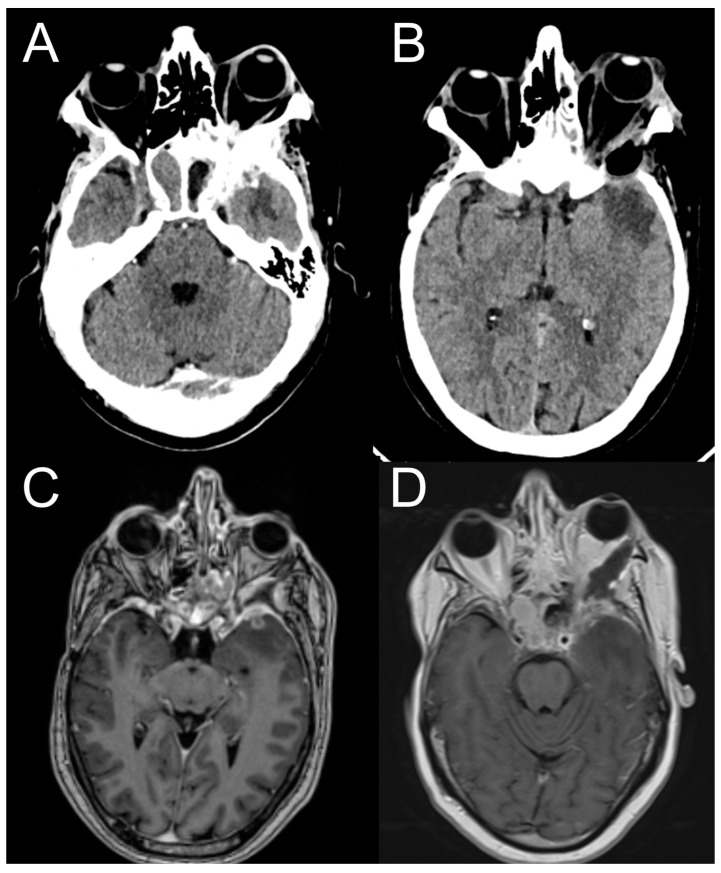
Pre-operative brain CT and MRI (A,C)show orbital infiltration by the primary tumor involving the temporal dura and the temporal lobe. Post-operative brain CT and MRI (B,D) show orbital decompression and resection of the brain tumor.
